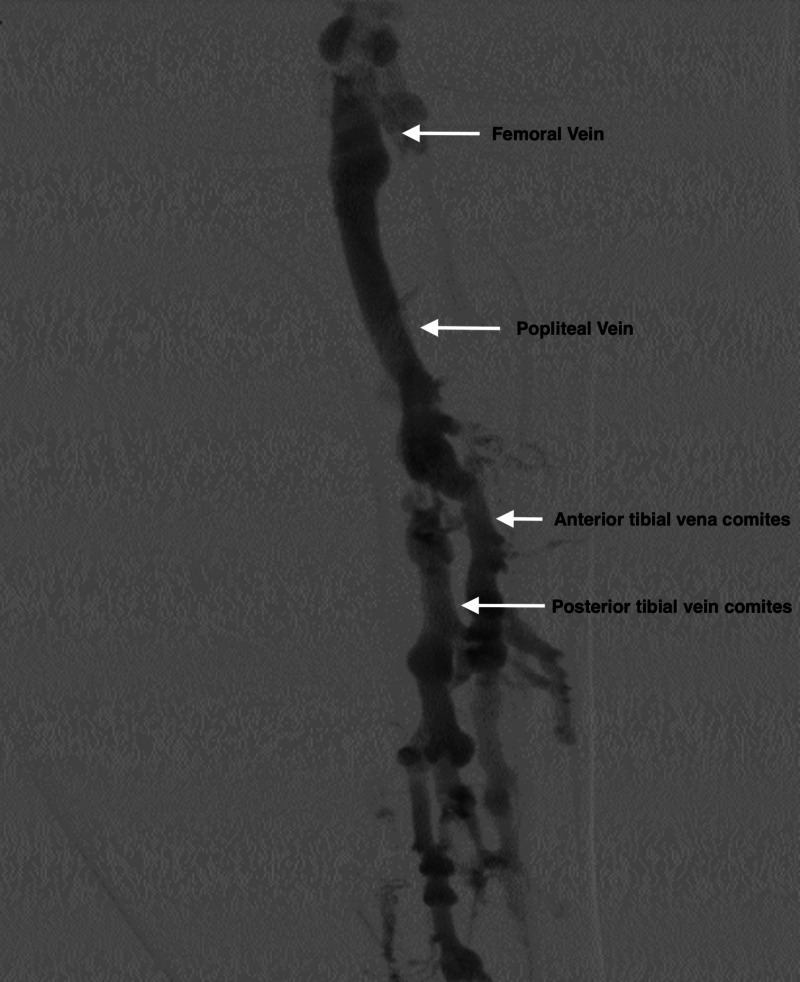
Left Lower Extremity Venogram Post-Intervention Venogram at 24 hours post-placement of an EKOS endovascular catheter in the left external iliac vein demonstrating overall clot burden in the tibial, popliteal and femoral venous system. EKOS endovascular catheters use ultrasound pressure with acoustic streaming and a thrombolytic agent to dissolve the thrombus. EKOS, EkoSonic
